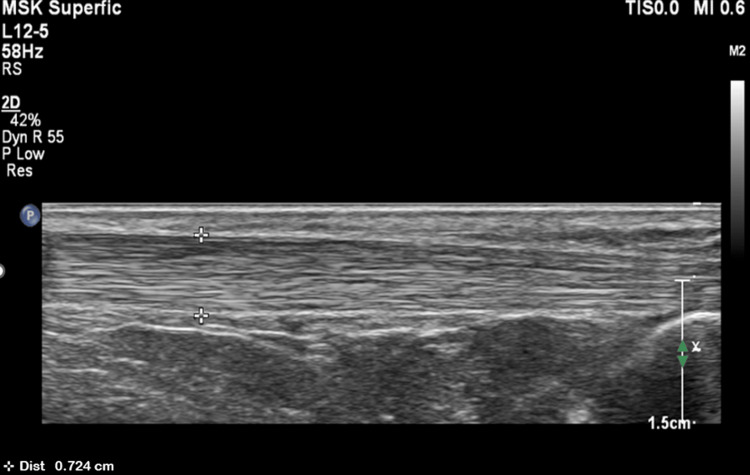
A greyscale ultrasound image showing a normal Achilles tendon using a linear probe (5-12 MHz)
